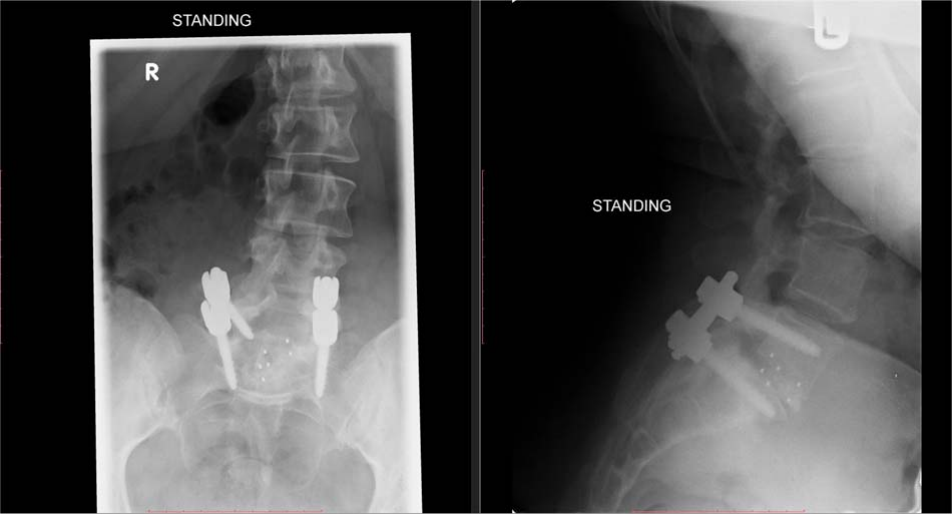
Postoperative X-ray of the lumbar spine performed in January 2017, showing the implants in place.
